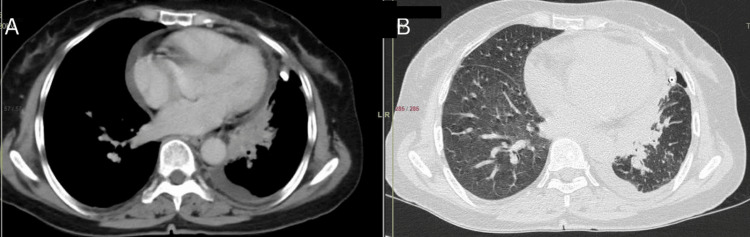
CECT thorax A: large heterogenously enhanced, ill-defined soft tissue attenuation of size 7.5 x 5 x 3 cm is noted in the left hilar and peri-hilar regions, with marked narrowing of the left upper lobe bronchus, and a left-sided pleural effusion is also seen; B: left hydropneumothorax with consolidation/mass in the hilar and peri-hilar regions CECT: contrast-enhanced computed tomography
